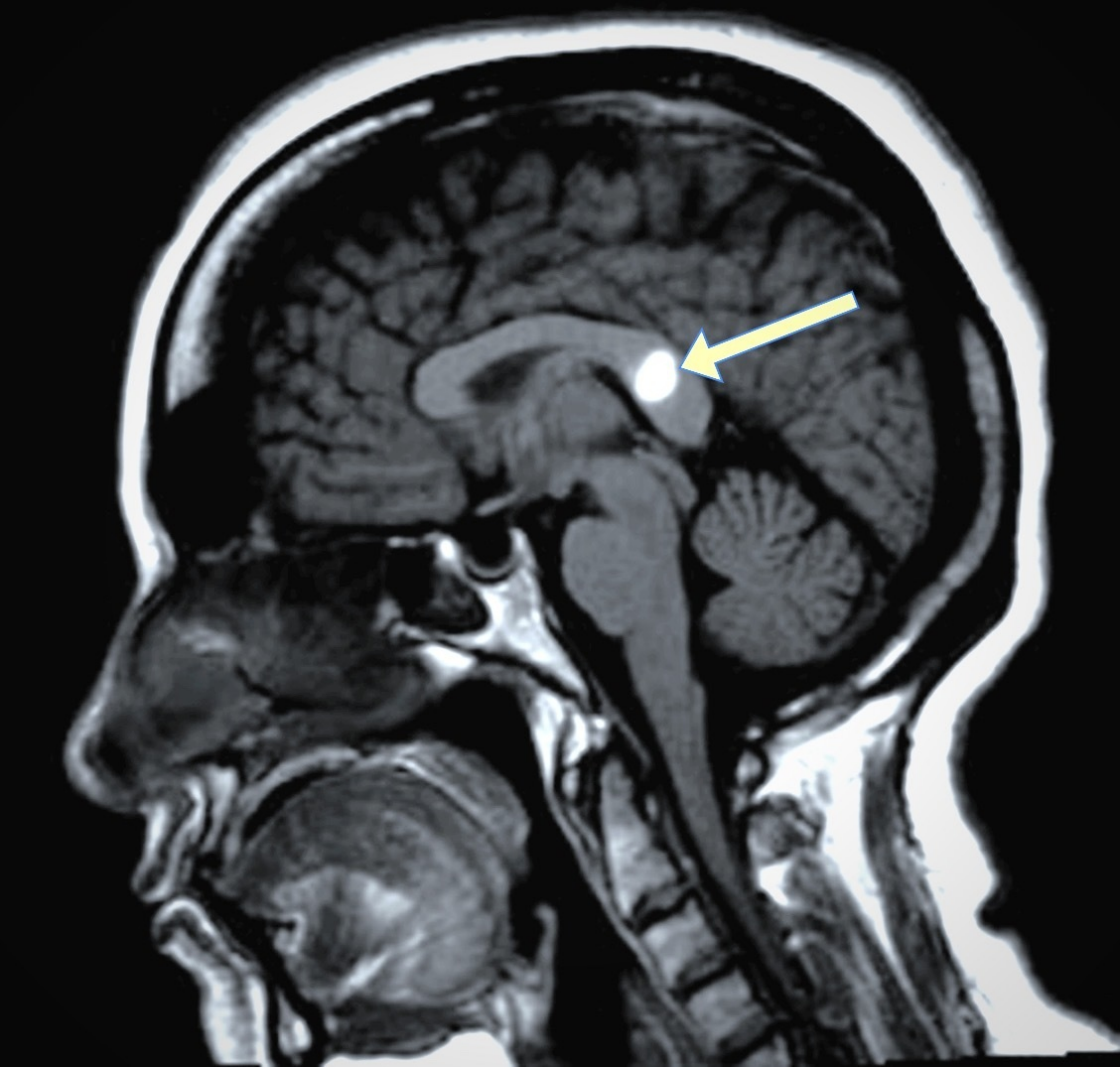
Brain magnetic resonance image (MRI T1W1) showing subacute hematoma of splenium of corpus callosum
